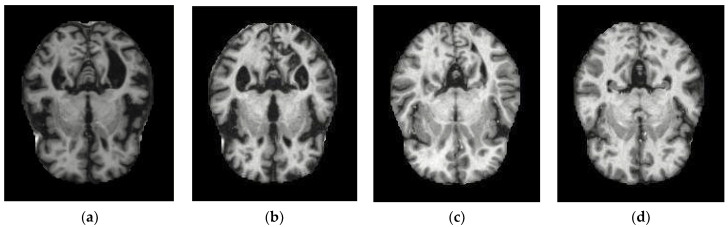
Alzheimer’s Disease MRI Dataset: (a) M.D, (b) Mod.D (c) N.D and (d) V.M.D.
